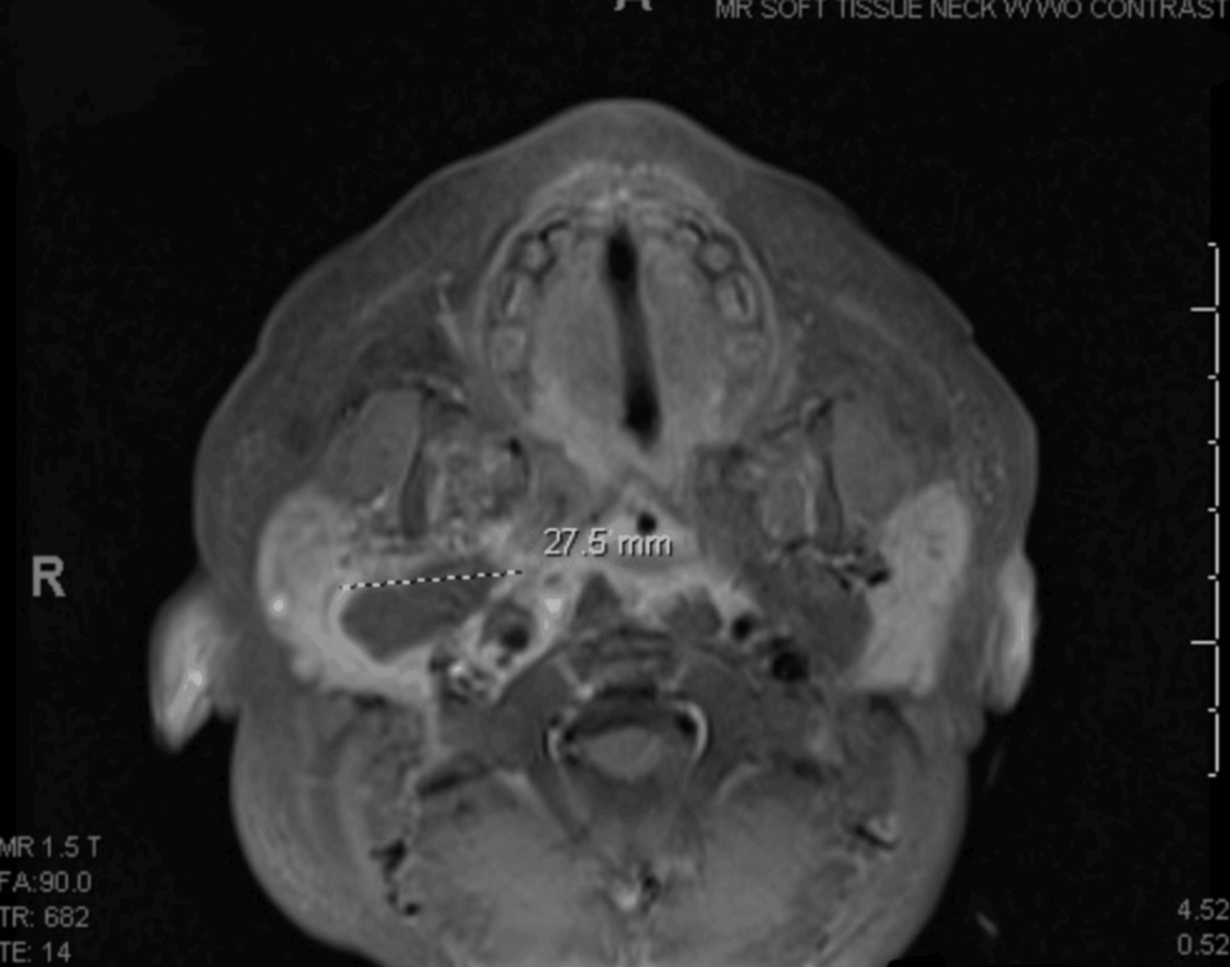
Axial post-contrast MRI of the neck demonstrating a 27.5 mm rim-enhancing fluid collection in the right parapharyngeal space, consistent with a deep neck space abscess. The collection involves the carotid and retropharyngeal spaces, with associated soft tissue edema and cellulitis.
